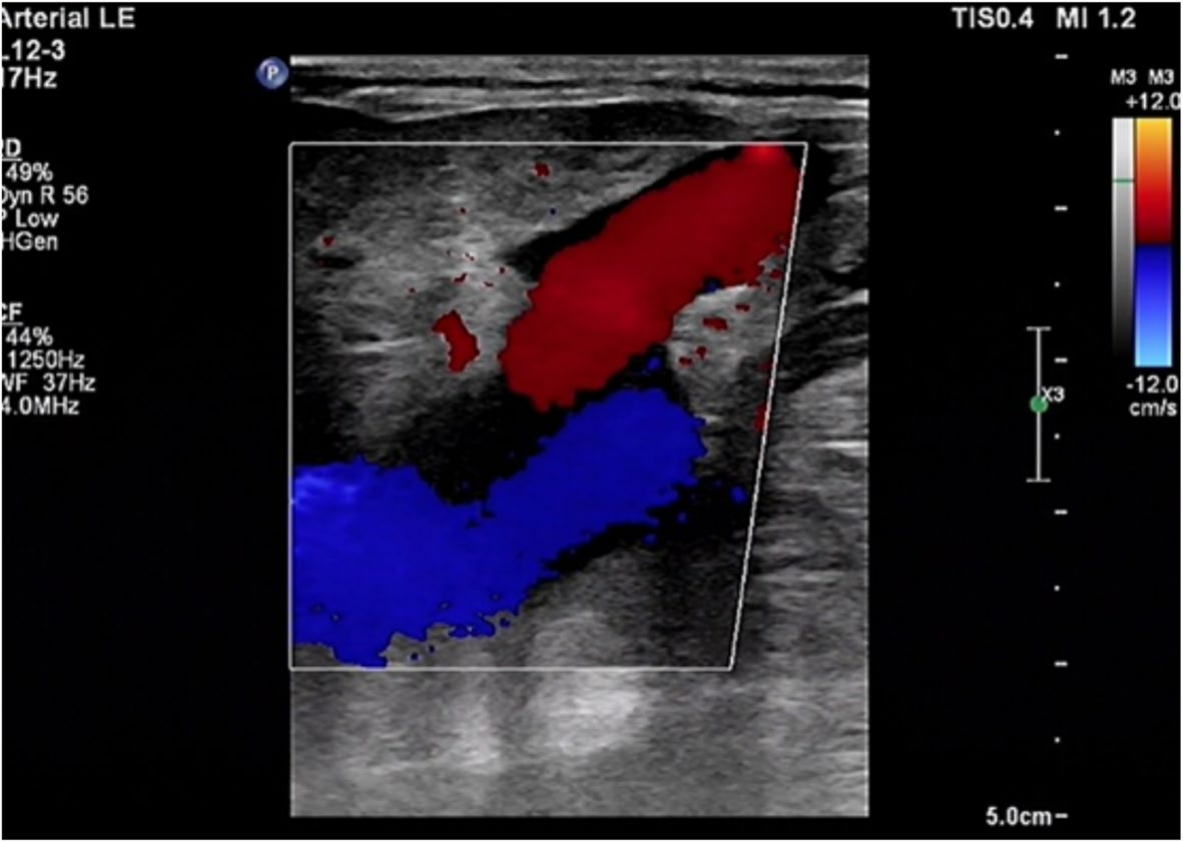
Color Doppler of the left sapheno-femoral junction showed continuous
